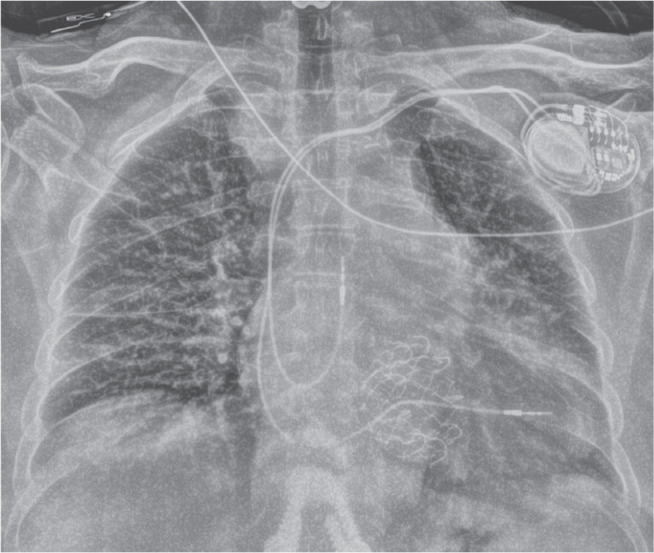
Frontal chest radiograph of a pacing lead that has been placed through a transcatheter tricuspid valve replacement.
